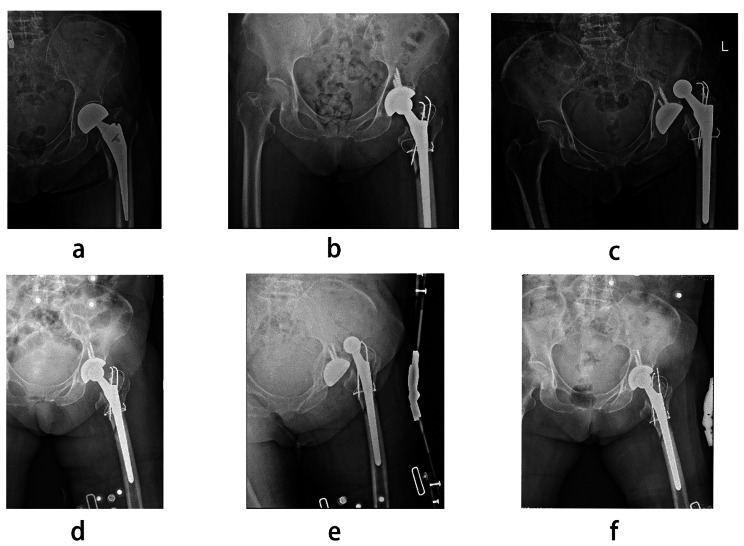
a A 76-year-old female who sustained a Vancouver type B2 fracture 21 months after hemiarthroplasty due to femoral neck fracture. b The patient underwent revision surgery with a nonmodular prosthesis, but postoperative pelvic anteroposterior X-ray showed that FO was not restored. c and d The patient had hip joint dislocation 1 month after revision surgery and received closed reduction. e and f The patient had redislocation of the hip joint 1 week after closed reduction and received closed reduction
